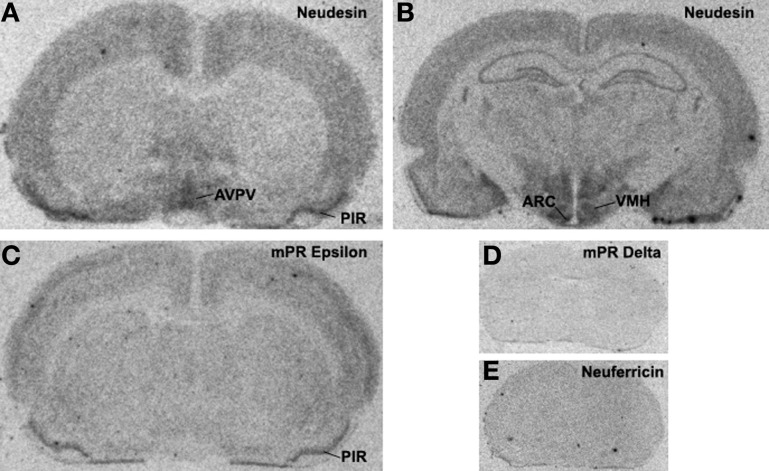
X-ray film autoradiograms showing results of *in situ* hybridization studies. Twelve-micron coronal frozen sections were hybridized to ^33^P-dATP end-labeled antisense deoxynucleotide probes for neudesin (Panels A and B), mPRε (Panel C), mPRδ (Panel D) or neuferricin (Panel E). Hybridization was as described previously (Intlekofer and Petersen, 2011) and sections were placed against Kodak BioMax MR film (Rochester, NY) for 2 weeks.
